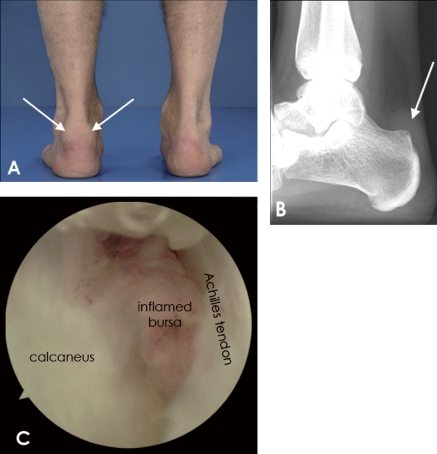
Clinical image (A) and radiograph (B) of a patient with a retrocalcaneal bursitis. The retrocalcaneal recess is obliterated by a chronic inflamed bursa (arrow). C. Endoscopic view of a patient with a retrocalcaneal bursitis.
